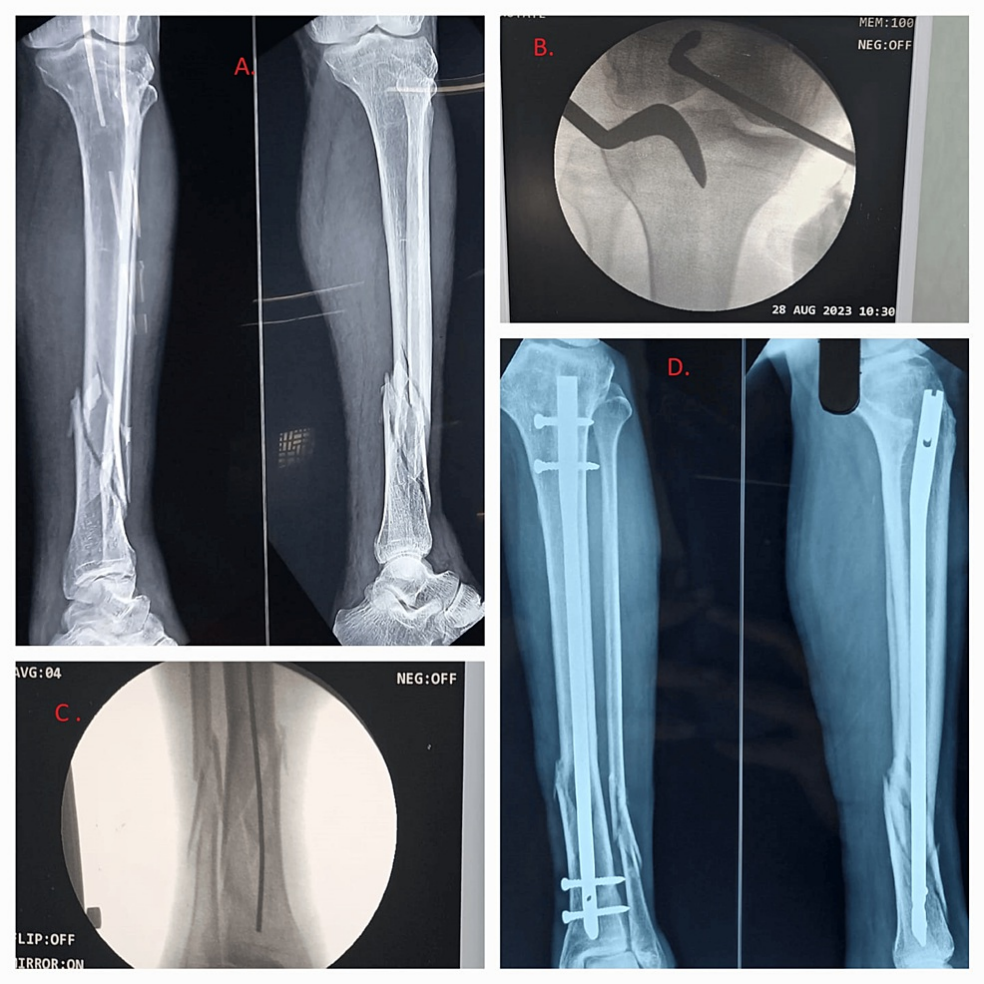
A 52-year-old male patient having distal tibia extra-articular comminuted fracture underwent tibia intramedullary nailing through lateral para-patellar approach in a semi-extended position. (A) Pre-operative radiograph showing distal tibia extra-articular comminuted fracture (AO/OTA 43-A3). (B) Anteroposterior view of entry being done with an awl passed through the lateral para-patellar approach. (C) Guide wire being passed through fracture site with closed reduction done in semi-extended position. (D) Immediate post-operative radiograph showing mild angulation. AO/OTA: Arbeitsgemeinschaft für Osteosynthesefragen/Orthopedic Trauma Association
